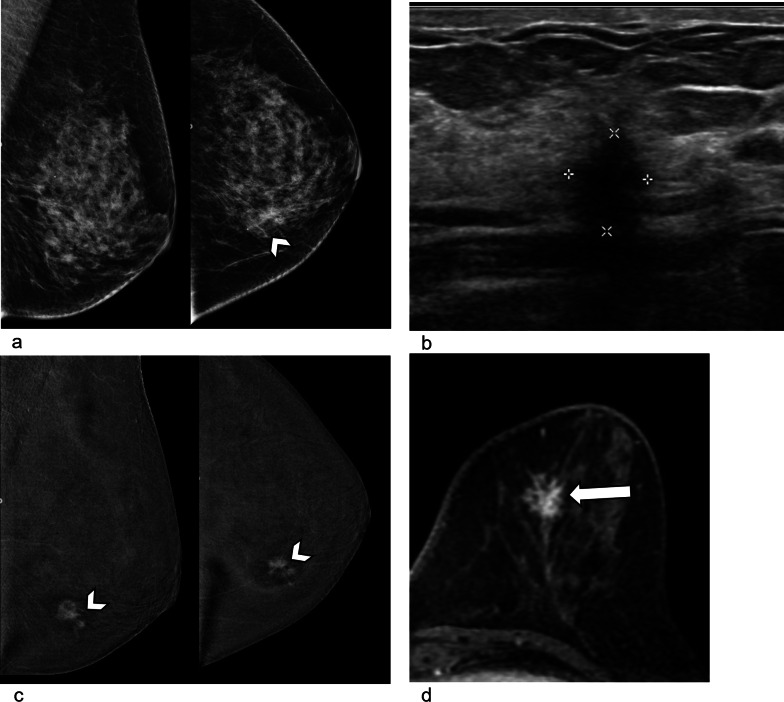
Primary ILC of the breast in a 61-year-old female who presented with a left breast lump. a Mammogram of the left breast shows focal asymmetry in the inner left breast on CC view (arrowhead), which is not well seen on MLO view. This corresponds to the palpable lump. b Ultrasound of the lower inner left breast demonstrates a corresponding irregular hypoechoic 1.0 cm nodule with posterior acoustic shadowing. This was proven on biopsy to be invasive lobular carcinoma. c Subtracted CEDM of the left breast shows the known ILC is enhancing with spiculated margins (arrowheads), with a tiny adjacent nodule not seen on ultrasound. The tumor measured larger on CEDM (1.6 cm) than on ultrasound (1.0 cm). d Axial contrast-enhanced MRI image of the left breast shows the spiculated, enhancing ILC (arrow), similar in size compared with CEDM. No other suspicious nodules were seen in either breast on CEDM and MRI
